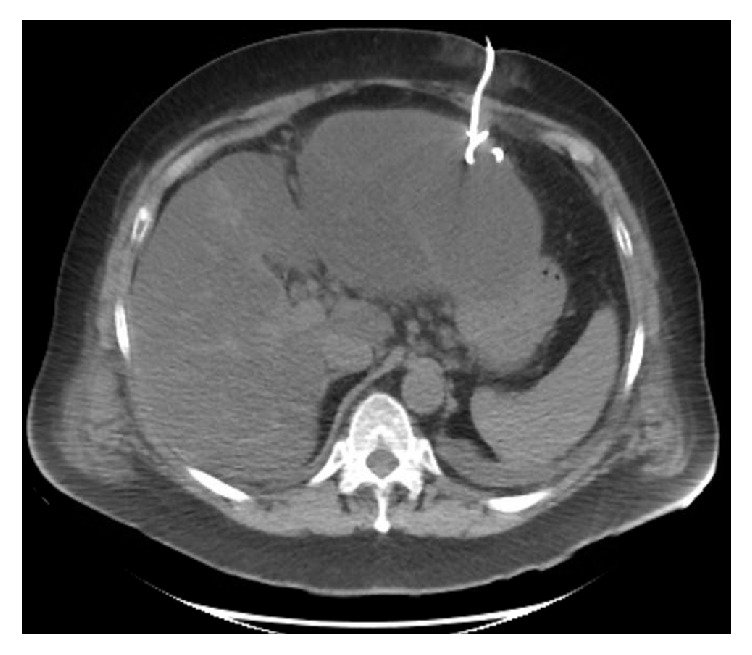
CT guided percutaneous drainage of subcapsular collection.
